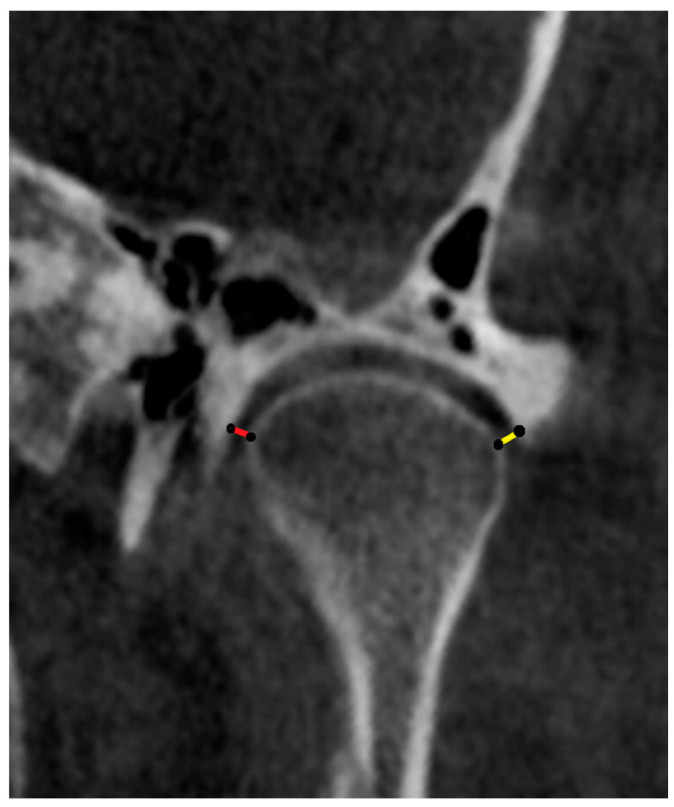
Coronal reformatted CBCT image of a patient showing measurement of the medial (MJS) and lateral (LJS) joint spaces. The measurements were carried out on the image in the coronal plane corresponding to the middle point of the condyle on the axial and sagittal plane on the slices with the largest condylar dimensions. MJS corresponds to the distance between the medial pole of the mandibular condyle and the medial wall of the glenoid fossa (red line). LJS corresponds to the distance between the lateral pole of the mandibular condyle and the lateral wall of the glenoid fossa (yellow line).
